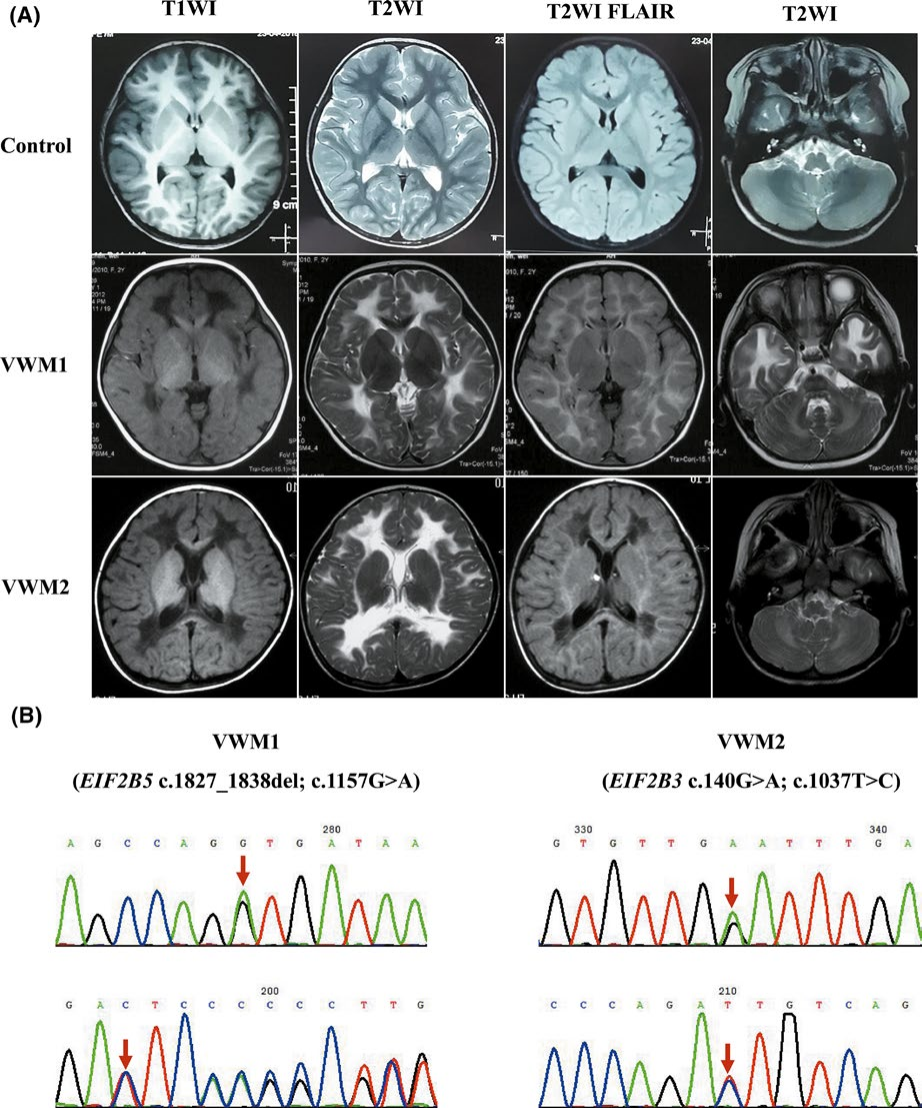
Features of the two VWM patients. a, Brain MRI of patients VWM1 and VWM2 and an age‐matched control, performed at the age of 4, 3, and 4, respectively: A, E, and I (T1WI); B, D, F, K, J, and L (T2WI); C, G, and K (T2 FLAIR). Brain MRI of both VWM patients showed symmetric abnormal signals on T1WI, T2WI, and T2 FLAIR in the white matter (WM), which was partially rarefied. b, Direct sequencing analysis of the genomic DNA from the two VWM patients’ iPSCs showed VWM1: *EIF2B5* c.1827_1838del (p. Ser610_Asp613del), c.1157G>A (p.Gly386Val); VWM2: *EIF2B3* c.140G>A (p. Gly47Glu), c.1037T>C (p. Ile346Thr)
